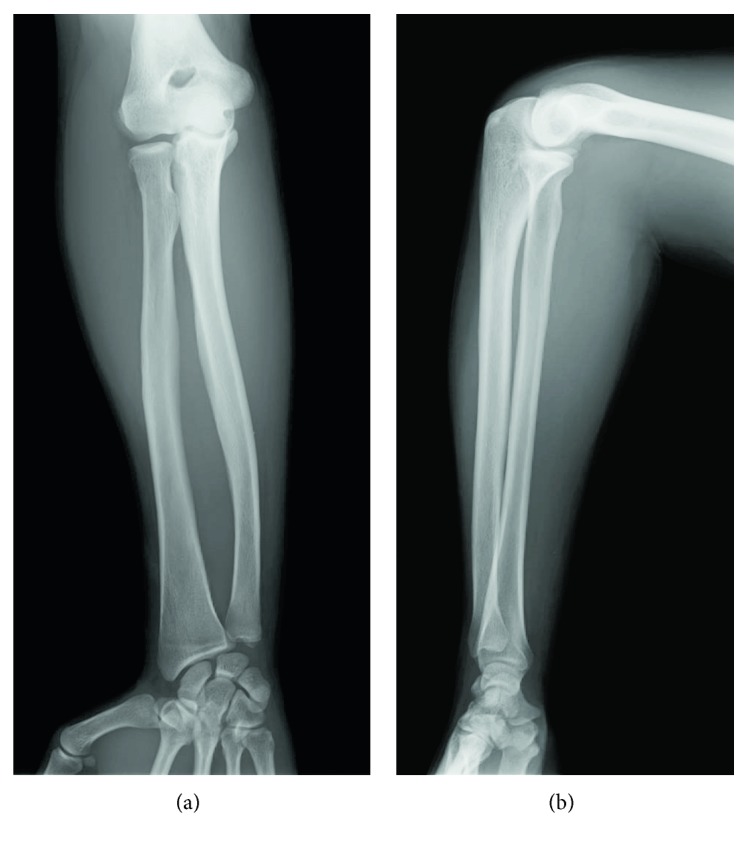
X-rays 21 years after surgery. (a) Front view. (b) Lateral view. The compatibility of the radiocapitellar joint was good, and no malunion was observed.
